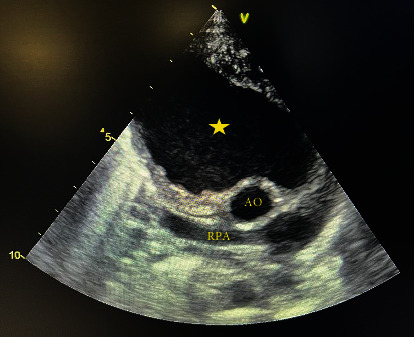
Transthoracic echocardiograph in suprasternal view showed a large pseudoaneurysm arising from the anterior wall of the ascending aorta. RPA: right pulmonary artery; AO: aorta.
